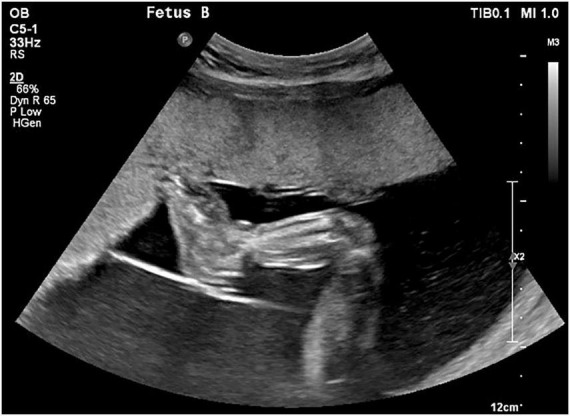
Ultrasonographic image of the fetal right foot. The 32-year-old mother had twin reversed arterial perfusion (TRAP) sequence and received microwave ablation for the acardiac fetus. Ultrasonography demonstrated an amniotic band encircling the fetal ankle, associated with subcutaneous tissue edema thickened to 0.6 cm.
